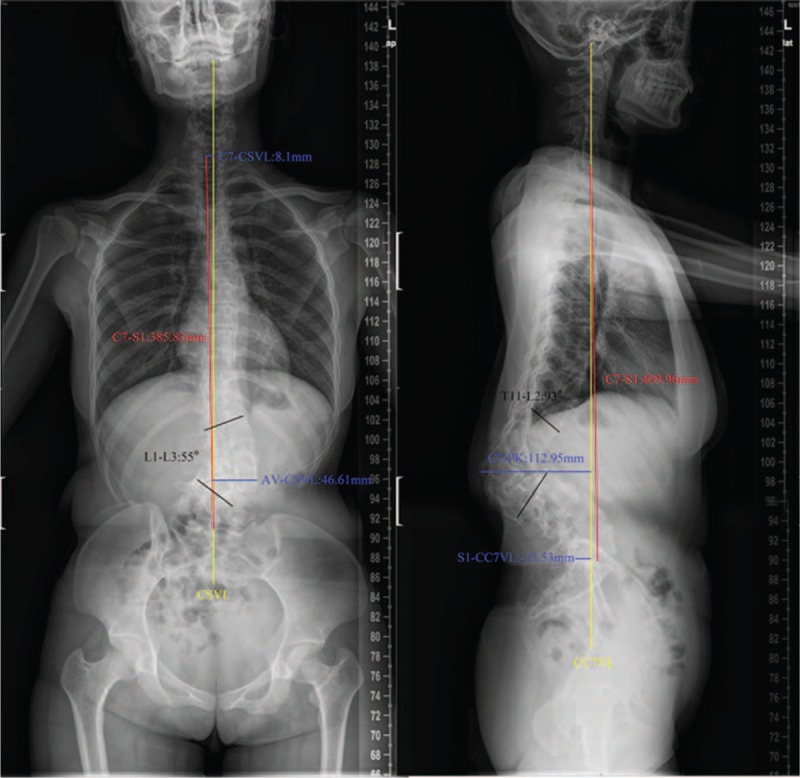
Lateral and anteroposterior X-ray, preoperation, lumbar kyphosis with a Cobb angle of 86° (L1–L2), and lumbar scoliosis with a Cobb angle of 55° (L1–L3).
